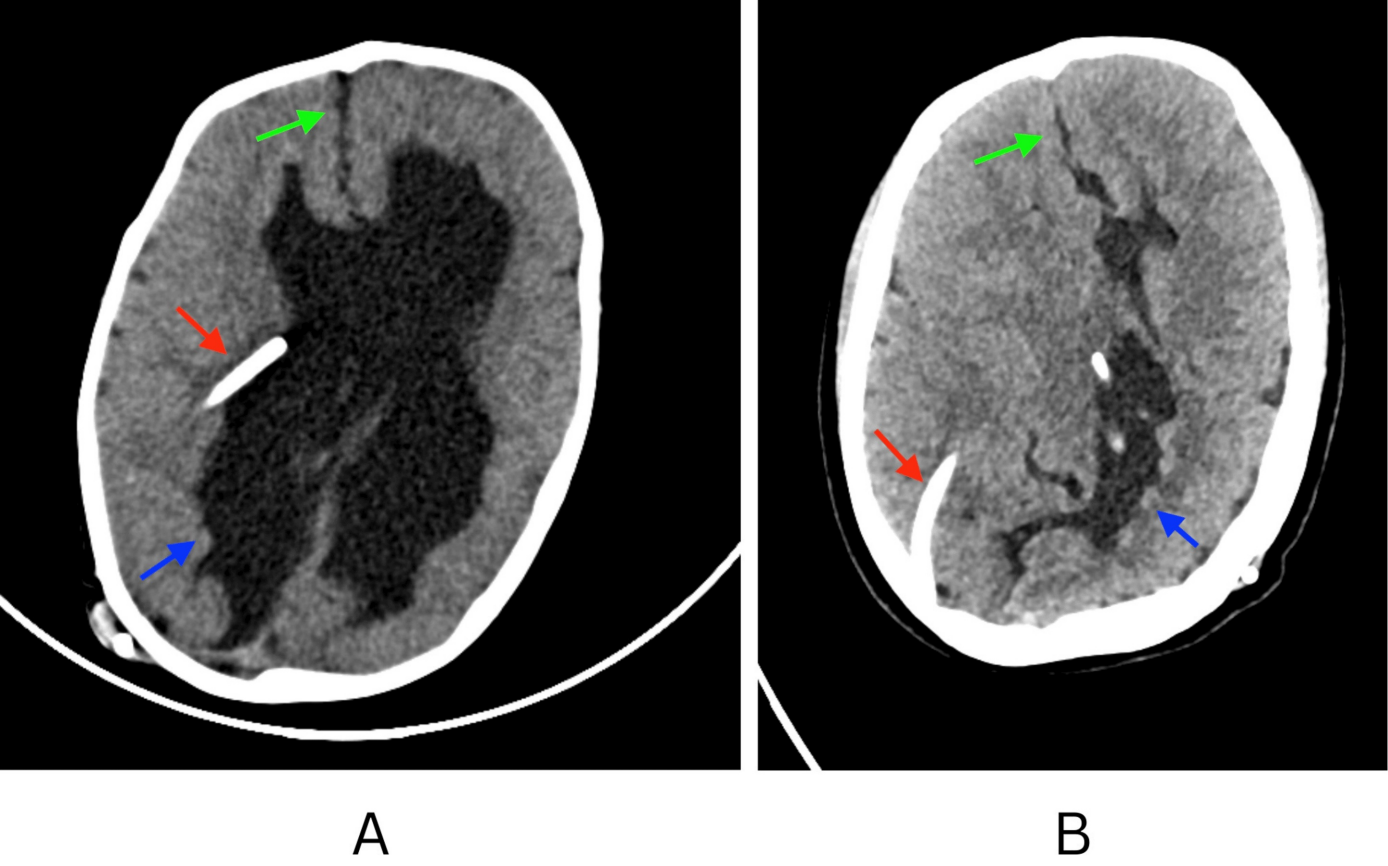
Non-contrast CT of the brain comparison between 2009 (A) and 2025 (B) demonstrating marked interval improvement in supratentorial ventriculomegaly over 16 years. Red arrows indicate the VP shunt catheter. Green arrows highlight midline findings consistent with corpus callosum agenesis with gyral interdigitation. Blue arrows indicate a right cerebral CSF cleft consistent with schizencephaly, persisting despite ventricular remodeling. CT, computed tomography; VP, ventriculoperitoneal; CSF, cerebrospinal fluid
